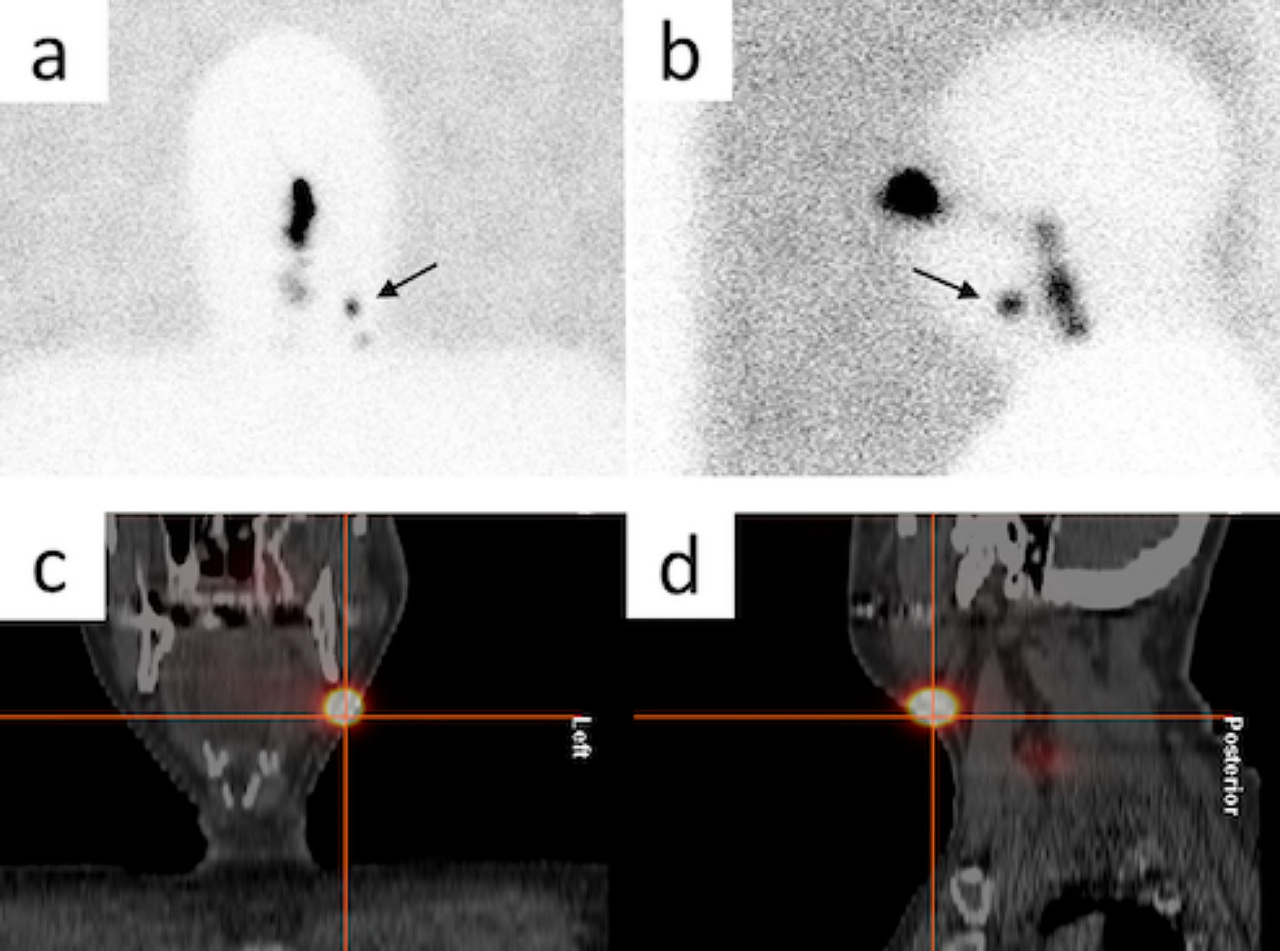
Sentinel node scintigraphy: (a) anterior and (b) lateral acquisition of static images, (c) and (d) SPECT/ldCT of the same SLN
